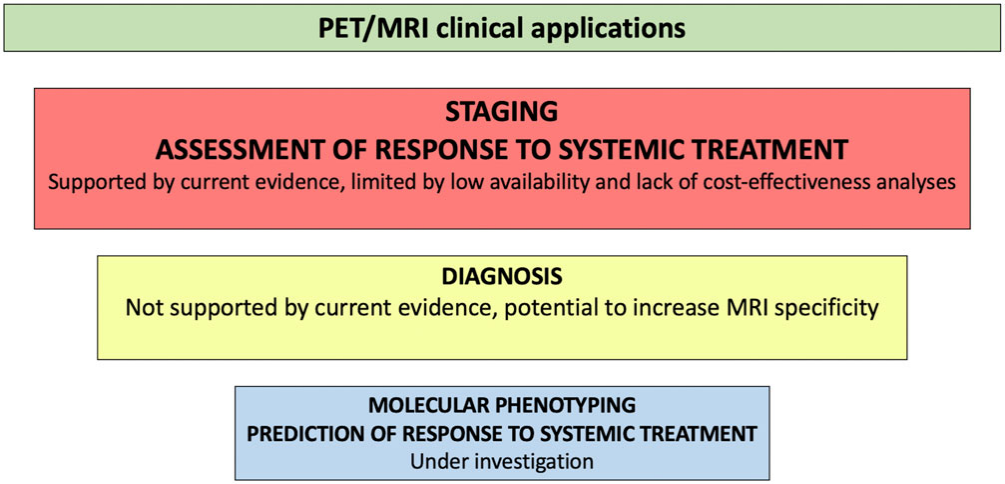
Clinical applications of hybrid PET/MRI in breast cancer in relation to their current evidence‐based status.
